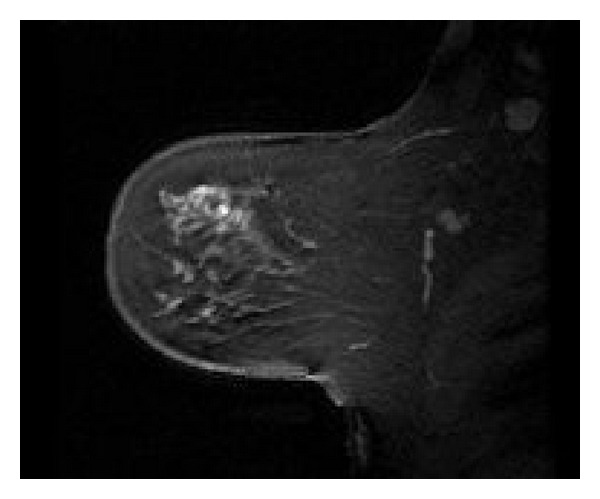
MRI appearance of recurrent DCIS.
